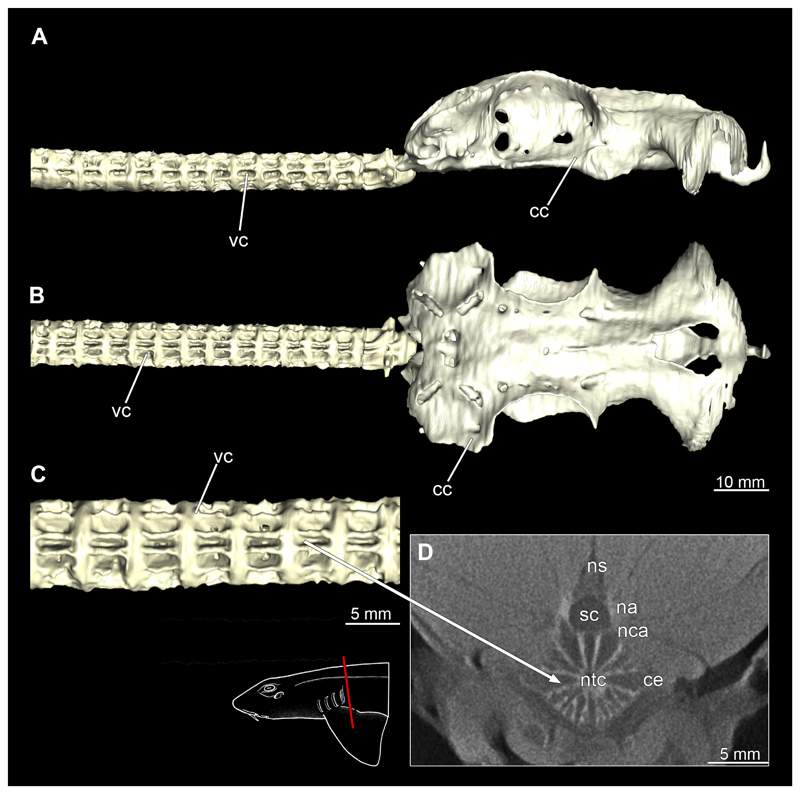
Chiloscyllium punctatum*, female: Vertebral column with chondrocranium as rendered surface. Arrow pointing to the longitudinal ribs running around the centre in the CT-image. A lateral, B dorsal, C detail lateral and D transversal cross-section through dorsal region (CT-image). Abbreviations: cc, chondrocranium; ce, centrum; na, neural arch; nca, neural canal; ns, neural spine; ntc, notochord; sc, spinal cord; vc, vertebral column.
